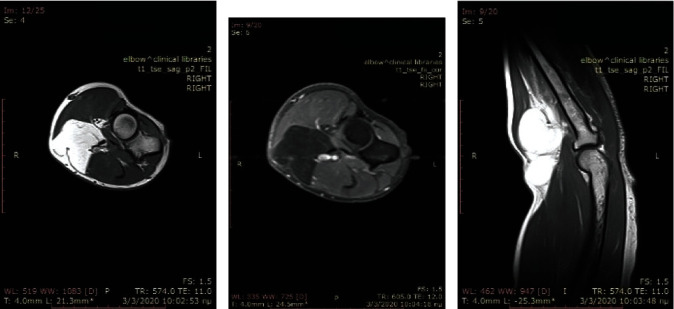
MRI images (performed with a 1.5-Tesla unit): (a) axial T1-weighted image; (b) axial T1 fat suppression; (c) sagittal T1-weighted image.
